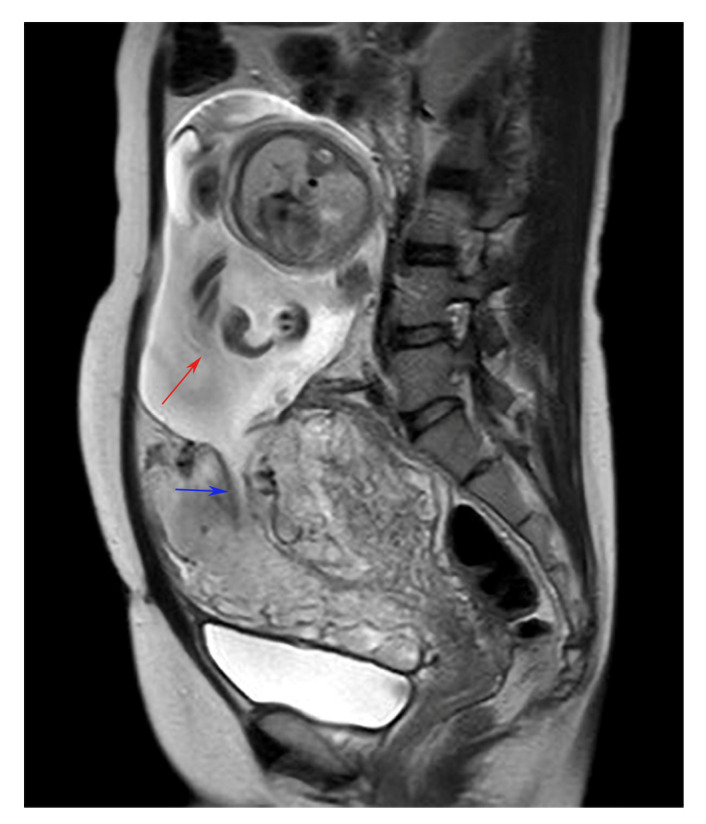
Pelvic MRI (sagittal) showing that the muscular layer of the uterine fundus was ruptured and the fetus had completely prolapsed into the abdominal cavity. The fetus is indicated by the red arrow, and the wound in the uterus is indicated by the blue arrow.
